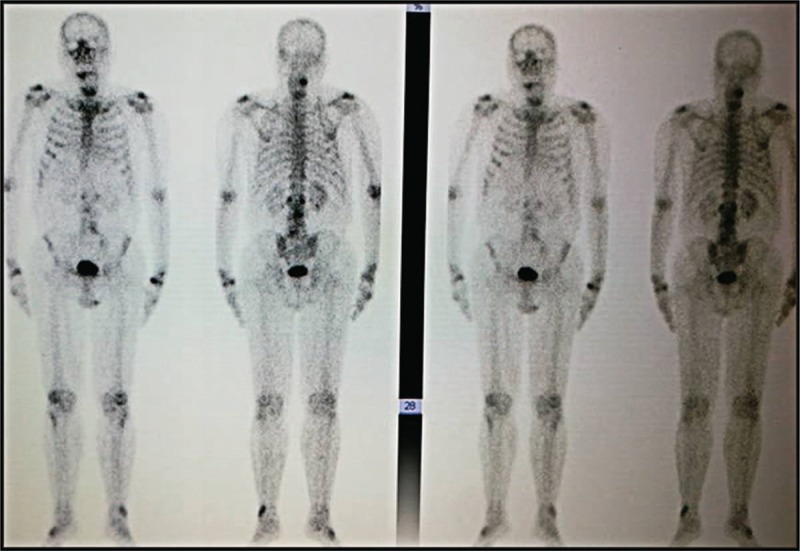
SPECT/CT scans show the disappearance of bone metastases.
